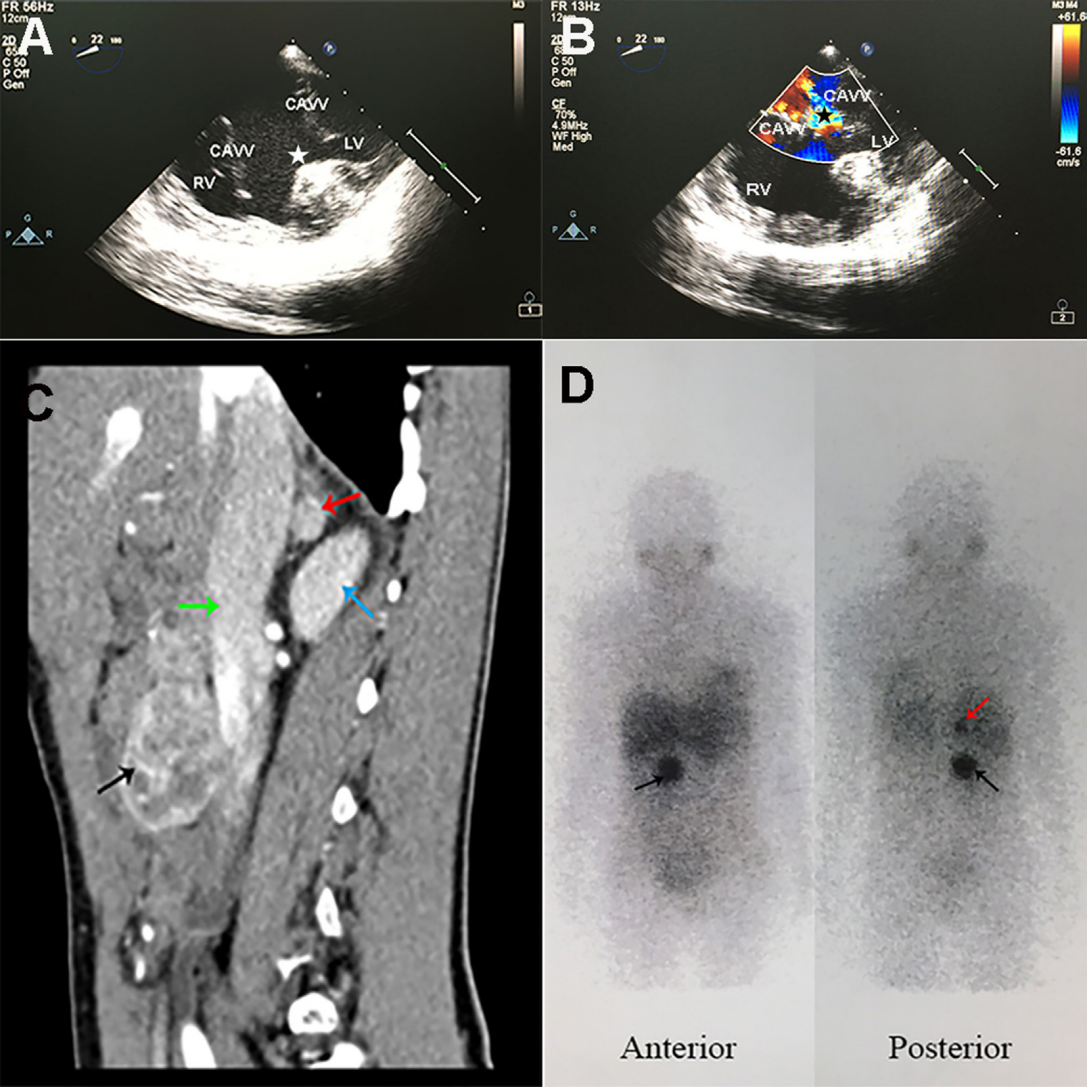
Specific radiographic imaging of the patient. (A,B) Trans-esophageal echocardiography showed functional single ventricle and severe common atrioventricular valve regurgitation. (A) CAVVs were open in cardiac diastole, and huge ventricular septal defect (white star) was observed. (B) CAVVs were closed in cardiac systole and regurgitation of blood into the ventricle (black star) was observed. RV, right ventricle; LV, left ventricle; CAVV, common atrioventricular valve. (C) Contrast-enhanced computed tomography sagittal reconstruction image of the abdomen showed a rounded mass (1.1 cm × 1.1 cm × 1.1 cm) in right adrenal gland (red arrow) and another mass (4.4 cm × 3.2 cm × 4.3 cm) with mixed density (black arrow) anterior to inferior vena cava (green arrow) at L2–3 lumbar level. The right kidney was showed with blue arrow. (D)
